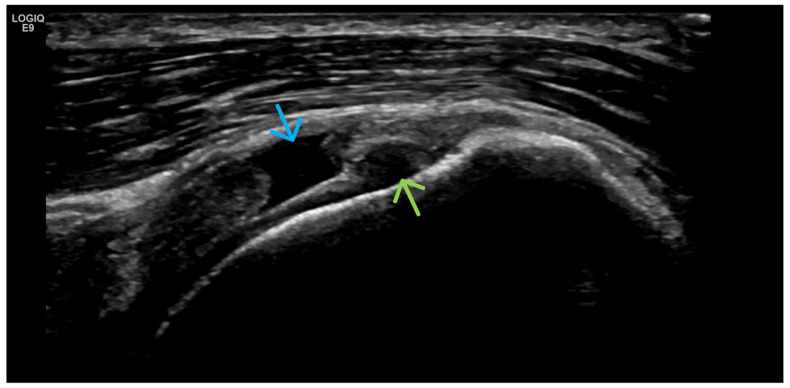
Anterior ultrasonographic scan of the shoulder joint of a patient with mechanical shoulder pain. Longitudinal US scan of the tendon of the supraspinatus muscle very clearly visualizes the partial ruptures of the tendon, which, through utilizing this imaging modality, are presented as anechoic zones—a partial rupture near the hyaline cartilage of the humerus (green arrow), as well as another in the middle of the bundles of tendon fibers (blue arrow).
